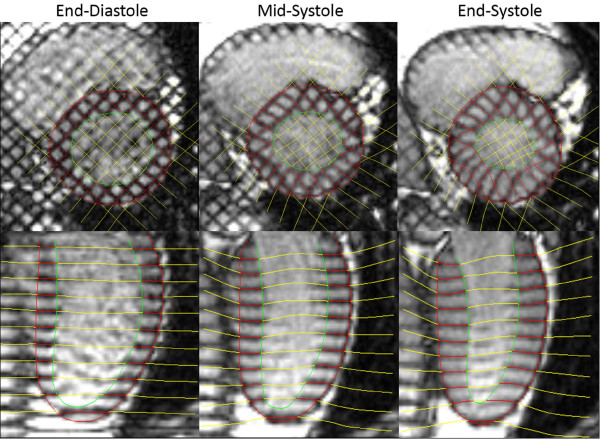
MRI Motion Tracking. Tagged MRI images of the left ventricle are shown at end-diastole, mid-systole, and end-systole. Images are acquired approximately every 30 ms throughout the complete heart cycle. Tag lines are semi-automatically tracked through systole, and displacements are computed using a previously described and validated method [20].
